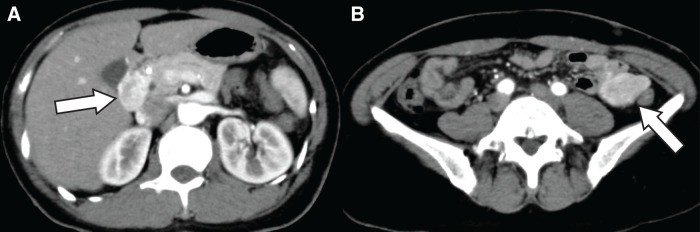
CE-CT findings. (A) A well-defined borderline mass 3.0 cm in size with clear contrast in the duodenal bulb near the pancreatic head (white arrow) is visible. (B) A mass 3.4 cm in size with a similar contrast effect is evident in the left lateral jejunum (white arrow). CE-CT, contrast enhanced-CT
